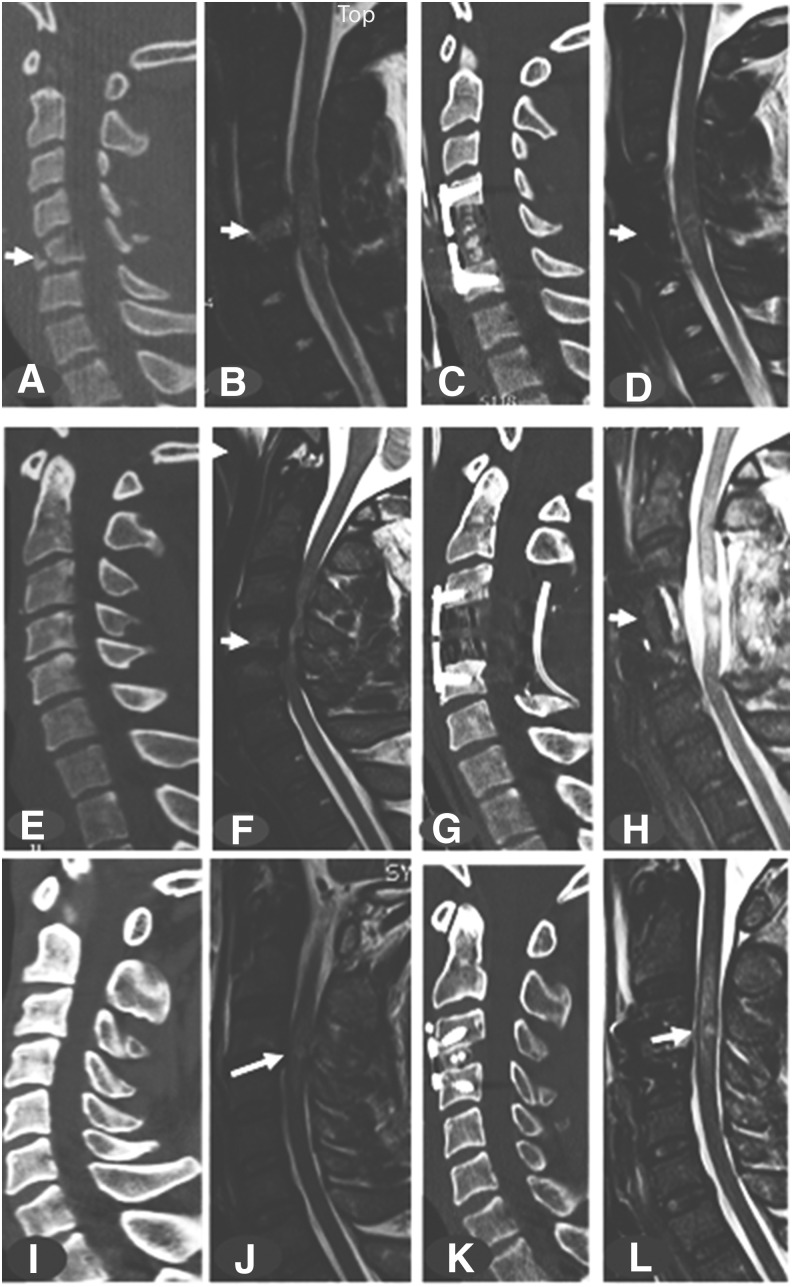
(A–D) Midsagittal computed tomography (CT) and magnetic resonance imaging (MRI) of a 19-year-old man involved in an automobile accident who was admitted 30 min later to the trauma resuscitation unit (TRU) with a C5 compression tear-drop fracture (arrow); American Spinal Injury Association (ASIA) motor score of 21, and ASIA Impairment Scale (AIS) grade B; intramedullary lesion length (IMLL) at admission was 27.8 mm. A C5 corpectomy was performed 6 h after the accident, which completely decompressed the spinal cord. MRI 34.5 h after surgery indicated an IMLL of 34.4 mm. One year following the accident, his ASIA motor score was 64 and he was AIS grade D. (E–H) Midsagittal CT and MRI of a 42-year-old man who had a mechanical fall and was admitted 30 min later to the TRU with from spinal stenosis and possible extension injury (arrow); ASIA motor score was 8 and AIS grade was A; IMLL at admission was 42.2 mm. He underwent C4 corpectomy and C3–C5 laminectomy with fusion 13 h after the accident, with complete spinal cord decompression. MRI 23 h after surgery indicated an IMLL of 30.9 mm. Six months following the accident, his ASIA motor score remained 8 and he was AIS grade A. (I–L) Midsagittal CT and MRI of a 53-year-old man who had a mechanical fall and was admitted 10.5 h later to the TRU with a C3/4 extension injury (arrow); ASIA motor score was 33 and AIS grade was C; IMLL at admission was 20.3 mm. He underwent discectomy and fusion at C3/4, 36 h after the accident, with complete spinal cord decompression. MRI 49.8 h after surgery indicated an IMLL of 49.6 mm. Fifty-seven months following the accident, his ASIA motor score was 91 and he was AIS grade D.
